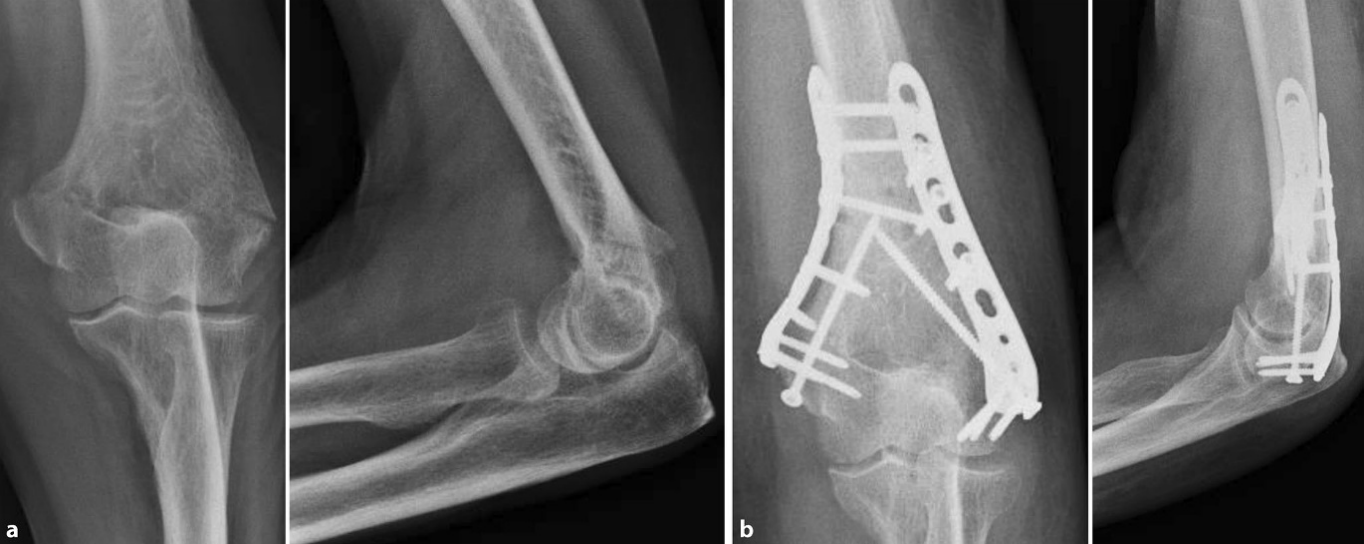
Extra-articular metaphyseal fracture, AO 13 A2 (60-year-old male patient). a Preoperative radiographs; b 90° double-plate construction (radial dorsal/ulnar) with anatomically preshaped 3.5 mm locking compression plates (LCP) and free cortical screws
